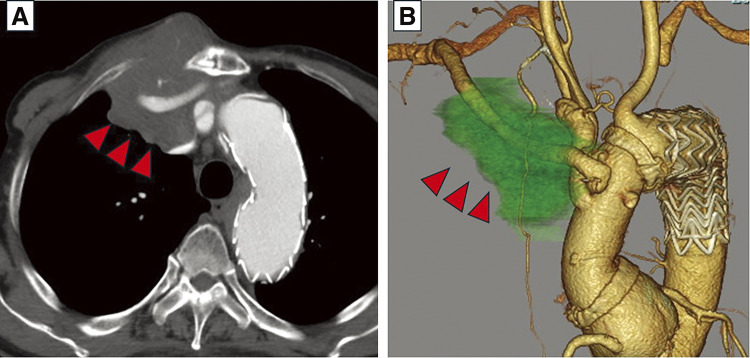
Fig. 1 Preoperative CT revealing a mass surrounding the graft to the right axillary artery. (A) Axial CT imaging showing tumor progression (indicated by red arrowheads). (B) 3D CT image highlighting the tumor with red arrowheads and light green coloring. CT: computed tomography
